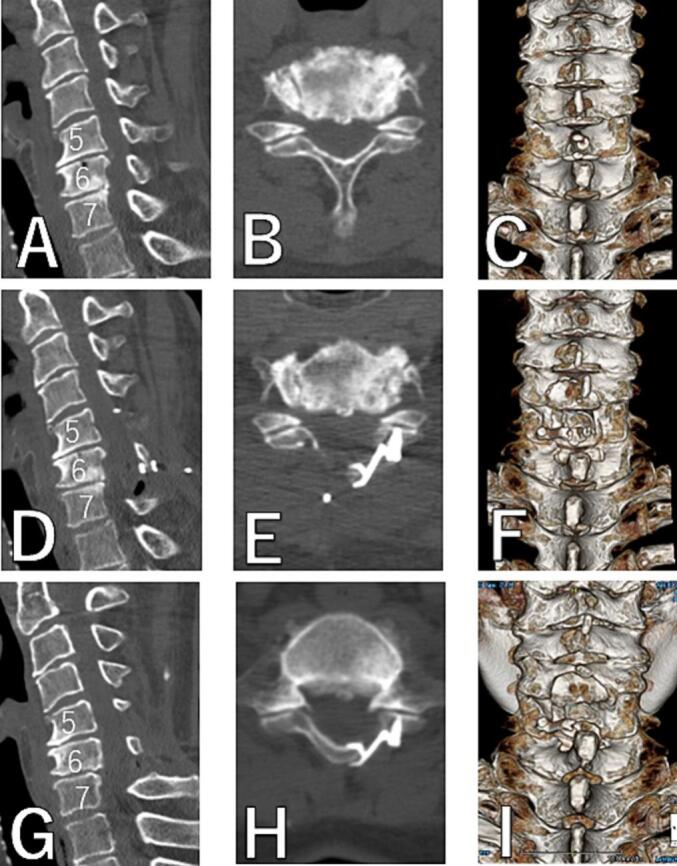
CT. A, B, C: preoperative. D, E, F: postoperative. G, H, I: 2 years postoperative. A, D, G: sagittal. B, E, H: axial at C5. C, F, I: 3D.
